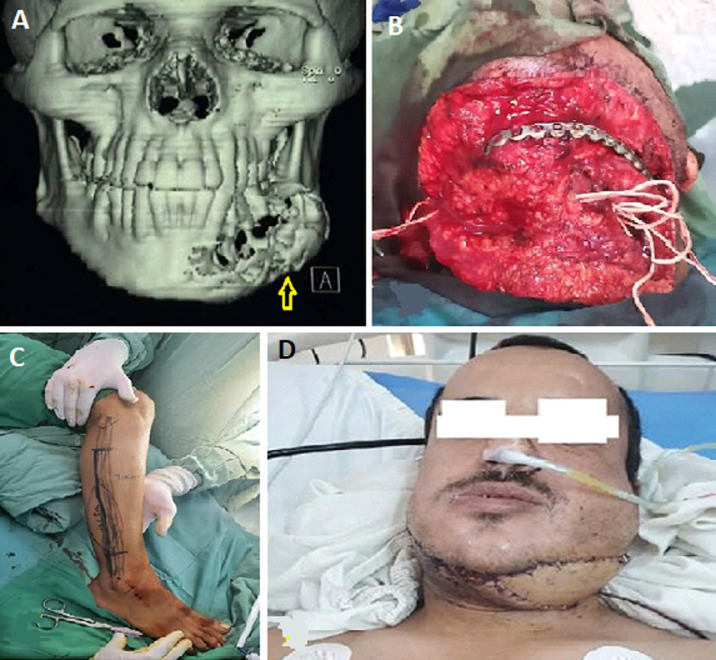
osteocutaneous fibula free flap: A) 3D computed tomography scan showing bony and soft tissue defects in the lower jaw (arrow); B) the intraoperative defect in the lower jaw; C) the fibula-free osteocutaneous flap design and preparation; D) the results of flap coverage after three weeks
